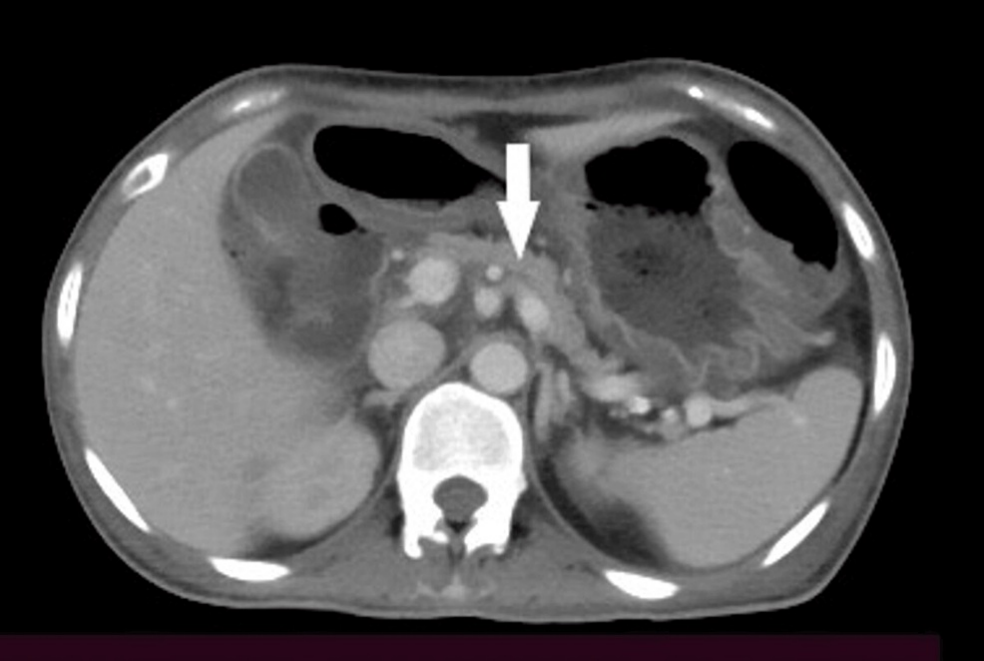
Arrow showing atrophied pancreas on CECT abdomen CECT: Contrast-enhanced computed tomography.
